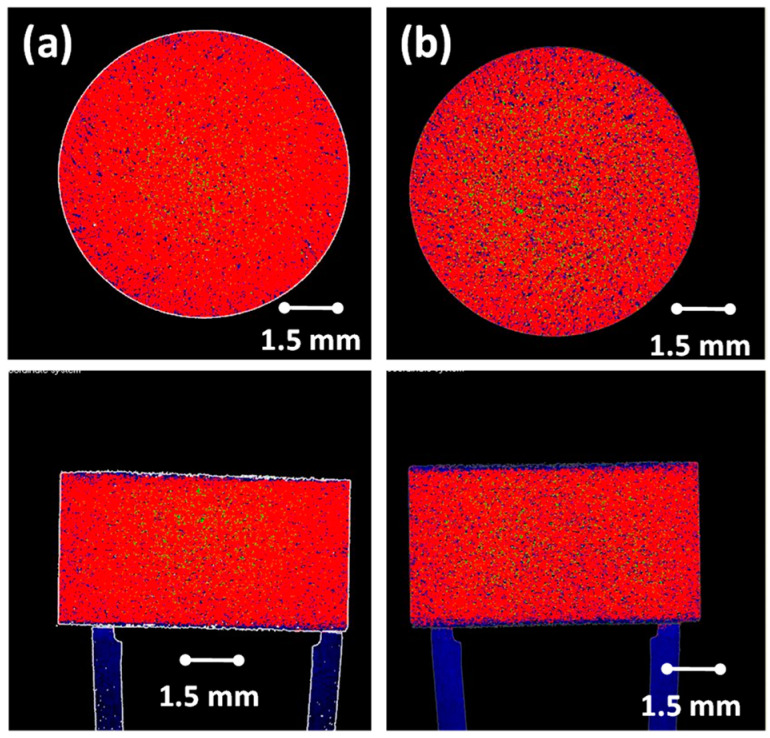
X-ray micro-tomographic images of sagittal and transverse sections through (a) CR and (b) DC formulation compacts. Images depict the homogeneity of the formulation mix.
